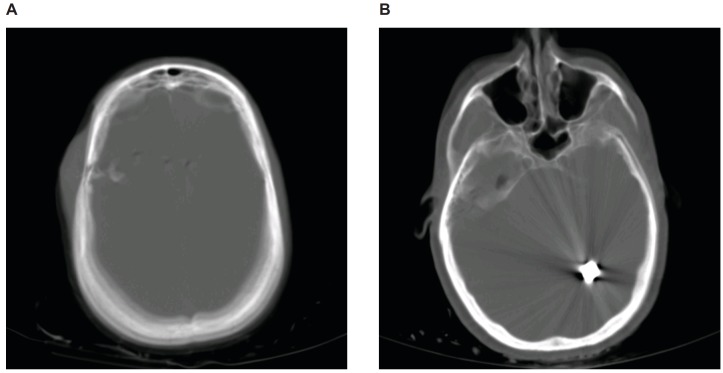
CT study of the head of the gunshot wound on admission (A) A comminuted fracture of the right parietal skull with displacement of the bony fragments is visualized. There is also a 2.2 centimeter displacement of the fragments into the right frontal temporal region. Soft tissue trauma with subcutaneous hematoma is also visualized over the lateral right parietal scalp. (B) A large bullet fragment is seen in the left parietal occipital region. Both panels are shown in the bone window.
